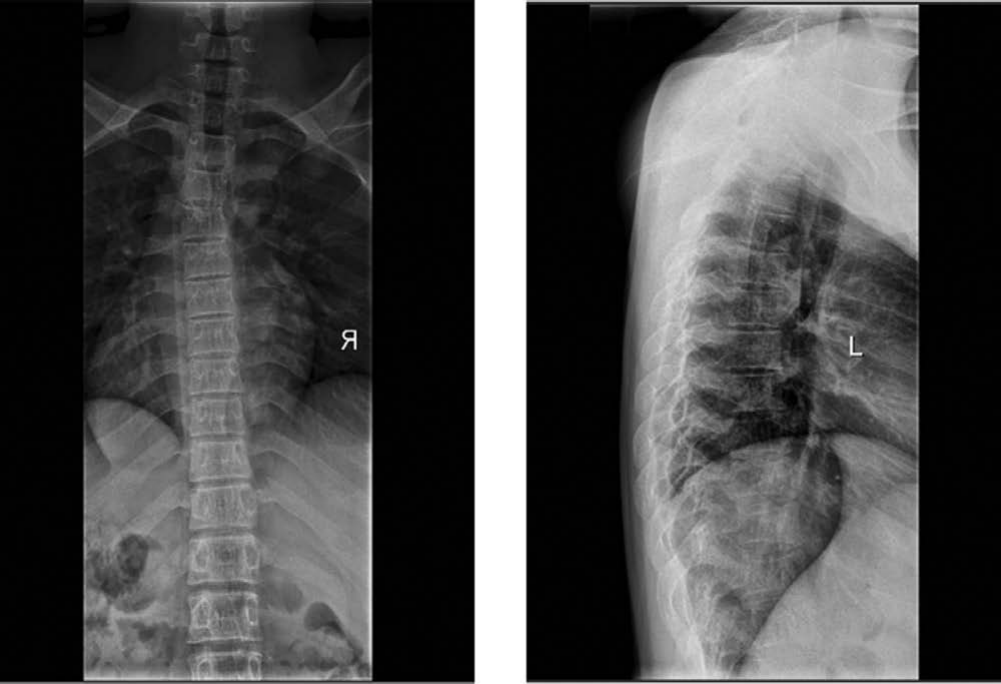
Magnetic resonance imaging (MRI) T2WI postoperatively showing relieved spinal canal compression of L5/S1 and S1/S2.
